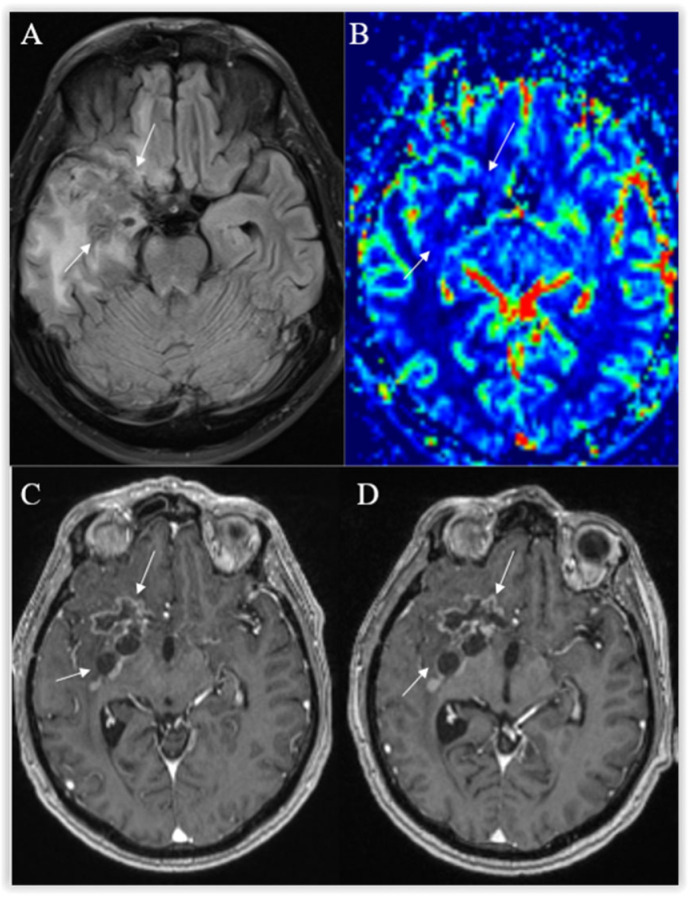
Radiation necrosis. Axial T2-FLAIR (A) and postcontrast axial T1W (B) images show heterogeneous an irregularly shaped area with cavitations, surrounded by moderate peripheral rim enhancement (arrows) and a wide T2-hyperintense zone of radiation-induced encephalopathy, with no significant mass effect. No frank hyperperfusion is seen on the DSC-based cerebral blood volume map (C). There is no significant change on a followup postcontrast axial T1W image (D) 11 months later.
